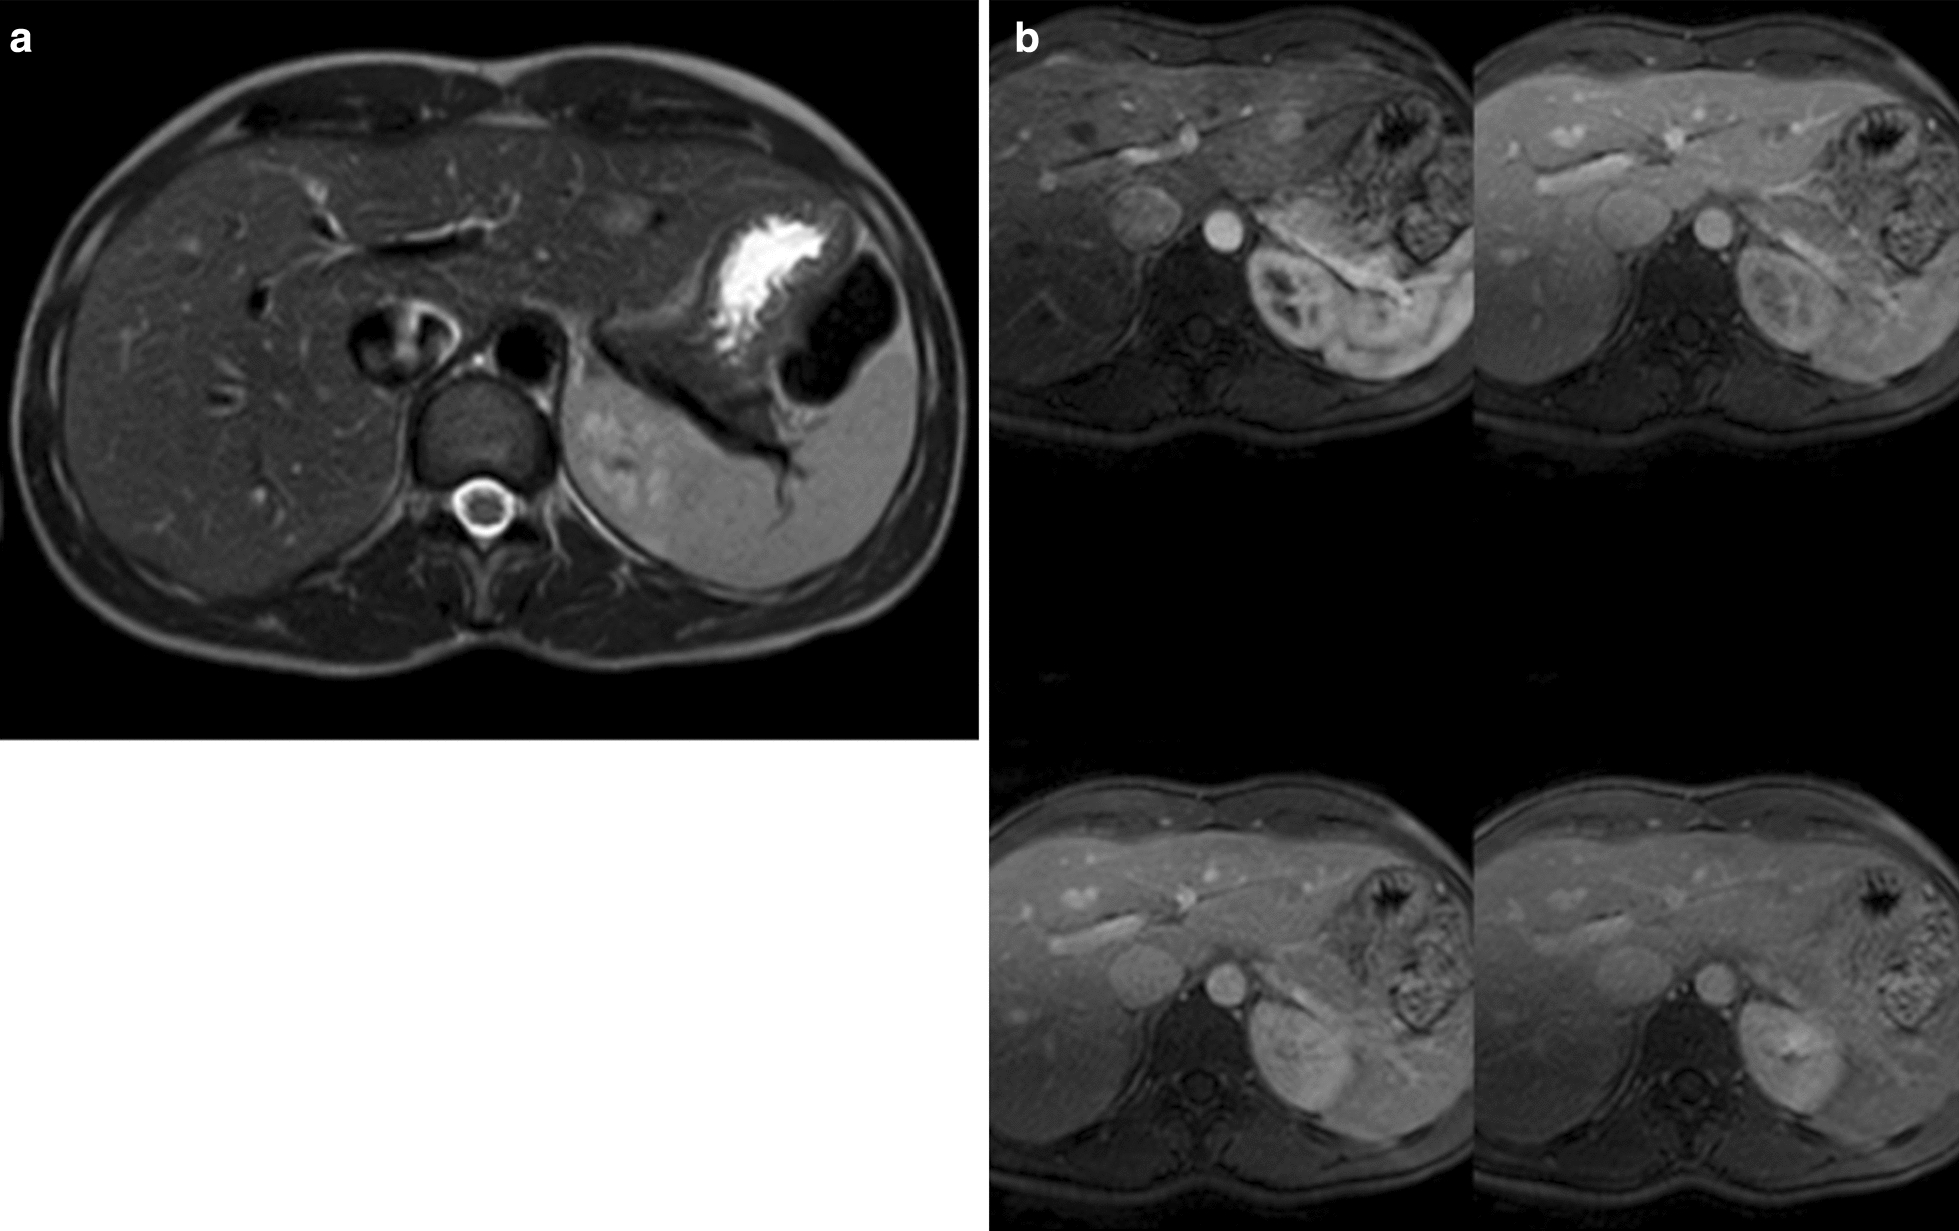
a, b Abdominal magnetic resonance imaging. In segment 2 adjacent to the left portal branch and below the suprahepatic branch, a focal lesion of 18 mm was identified. The lesion is hypointense in T1, moderately hyperintense in T2 (a), although with poorly defined edges and with clear diffusion restriction. In the dynamic study, it is a hypervascular lesion in the arterial phase that washes in the portal and late phases (b)
